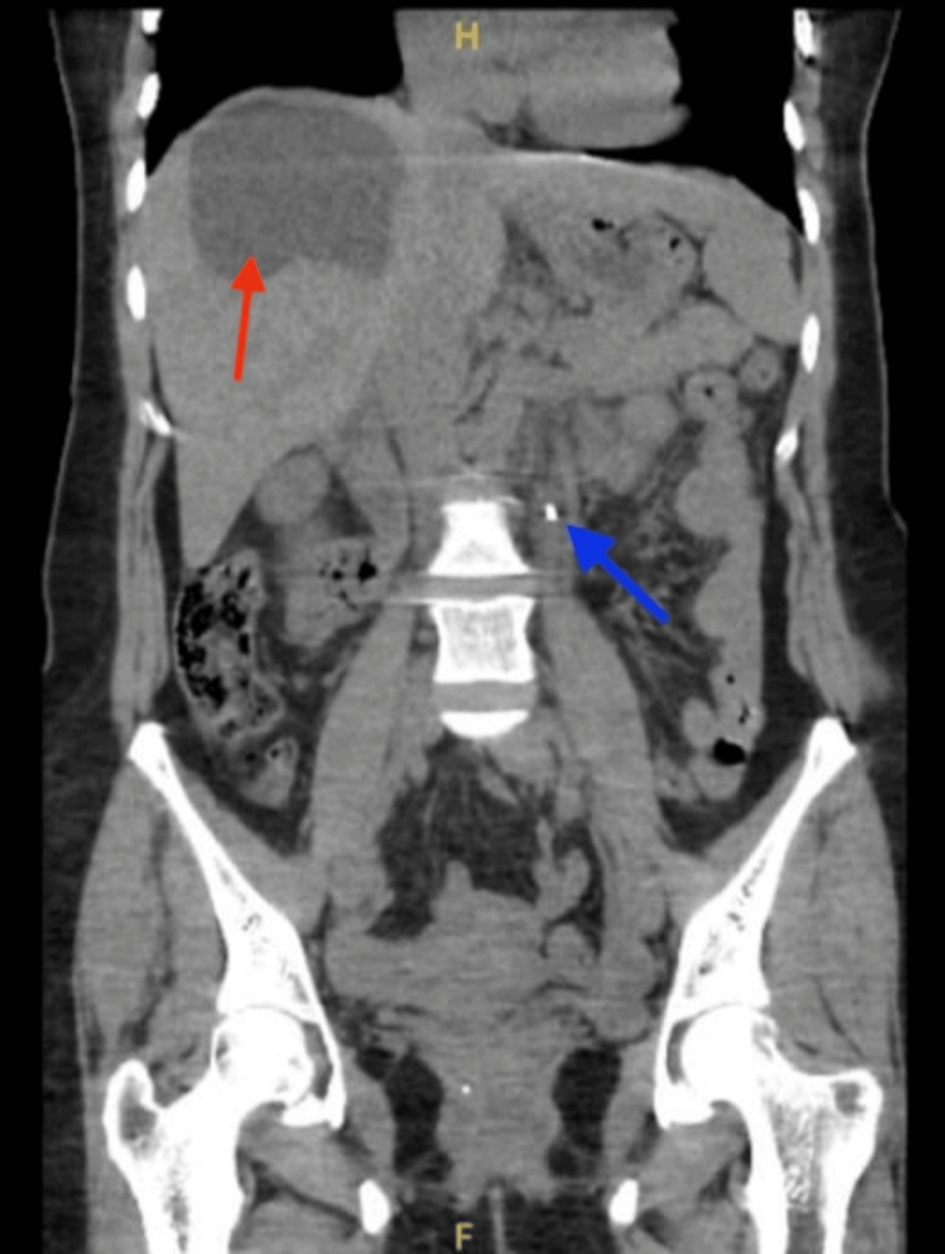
First Encounter CT-KUB (Coronal View) Showing a 5 mm Left Ureteric Stone (Blue Arrow) and Incidental Finding of a Large, Non-calcified Cystic lesion in the Right Hepatic Lobe (Red Arrow). CT-KUB: Computed Tomography of the Kidneys, Ureters, and Bladder
